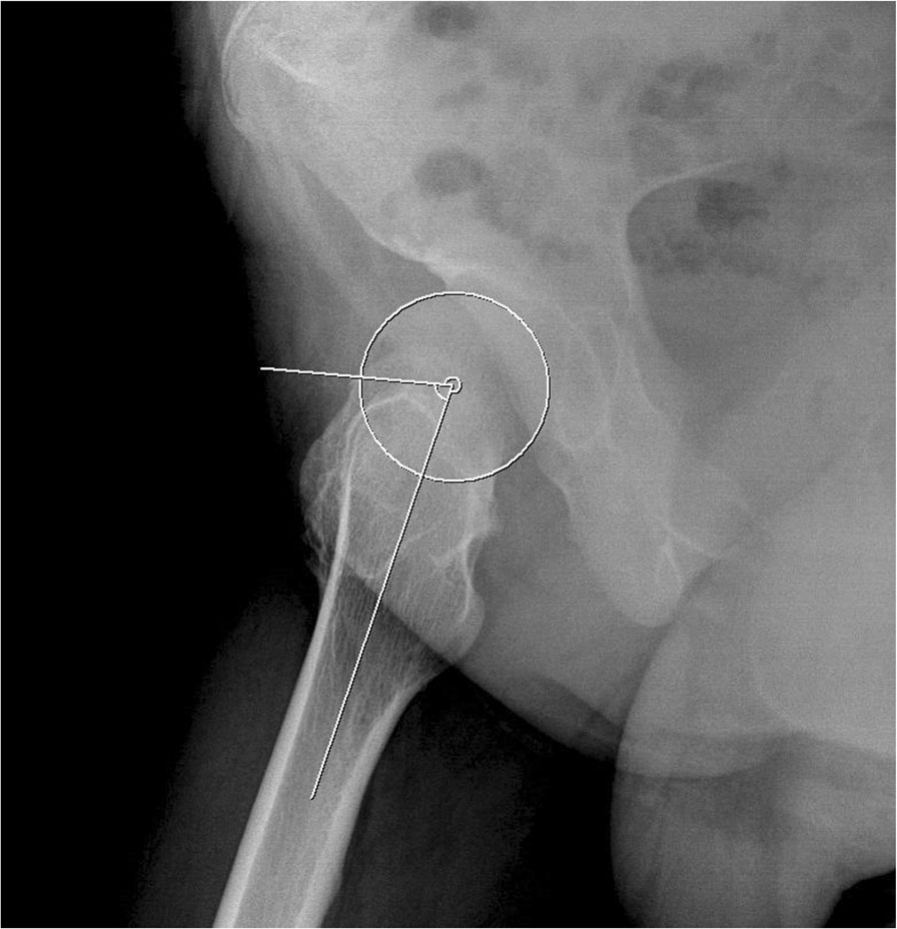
Measurement of the radiographic alpha angle. The alpha angle is determined by the angle between a line from the center of the femoral head through the middle of the femoral neck and a line through a point where the contour of the femoral head-neck junction exceeds the radius of the femoral head
